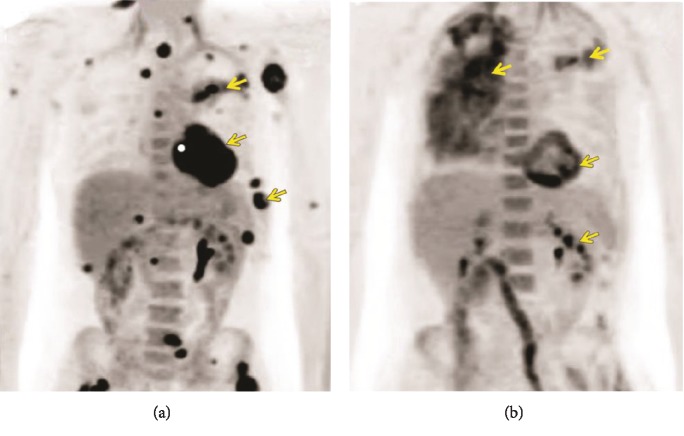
(a) Fluorodeoxyglucose positron emission tomography/computed tomography (FDG PET/CT) shows development of widespread hypermetabolic metastases (arrows). (b) FDG PET/CT shows more widespread metastasis involving the right lung and adrenal glands (arrows).
